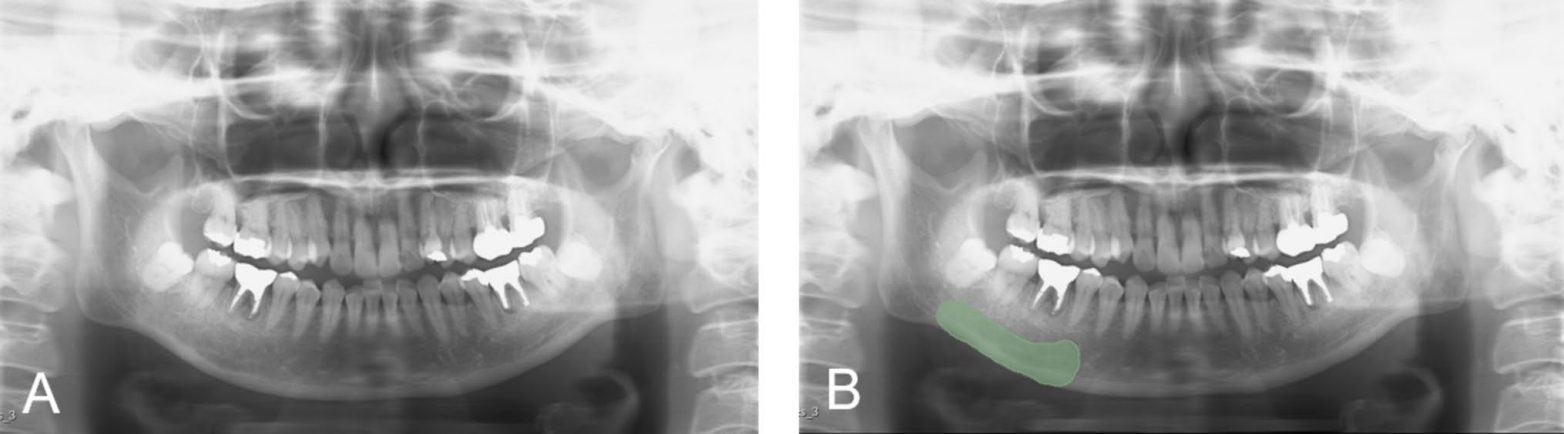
Example of segmentation using panoramic radiographs. A Original panoramic radiograph before treatment. B Segmentation of the mandible before treatment
